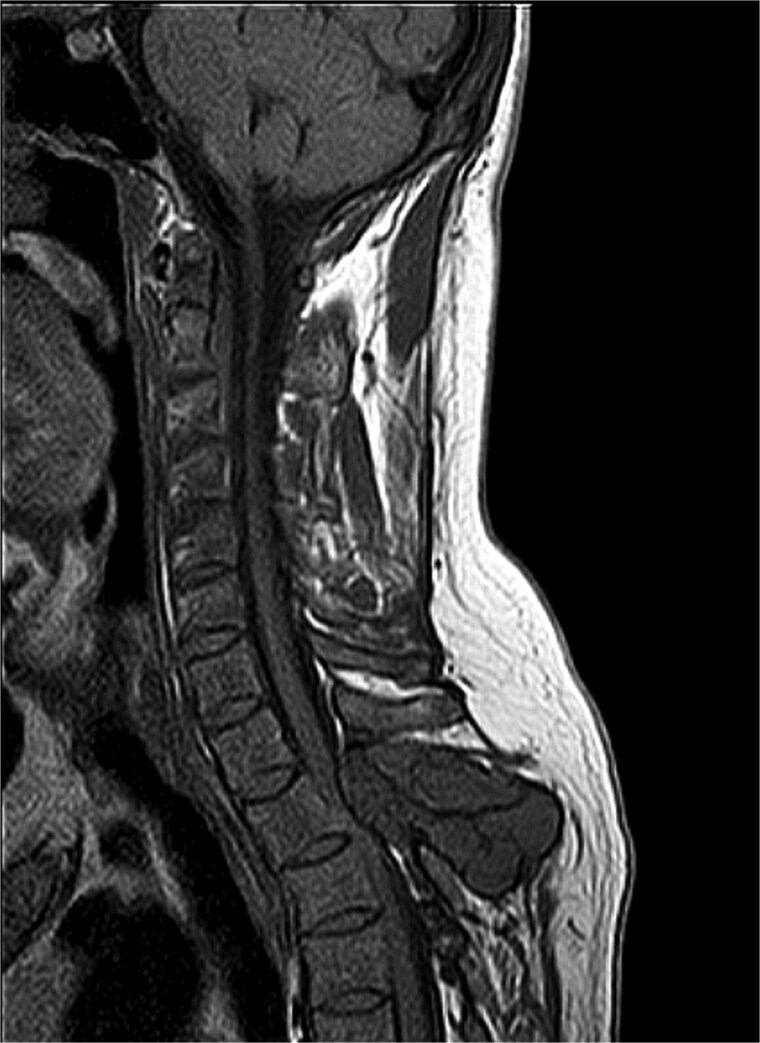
MRI thoracic spine: Extradural lobulated minimally enhancing lesions involving spinous process of T2 vertebra, right pedicle and lamina of T3 vertebra and posterior aspect of left T2 rib.
